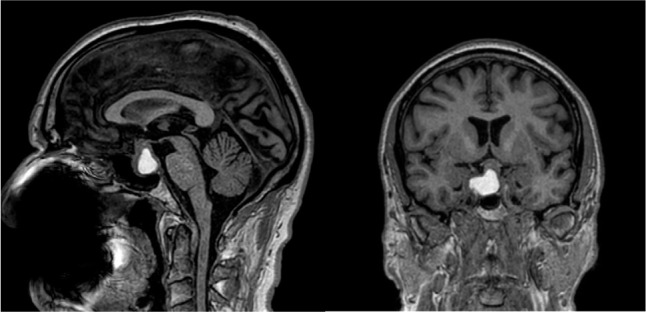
Sagittal and coronal views of T1 MRI without gadolinium showing an
